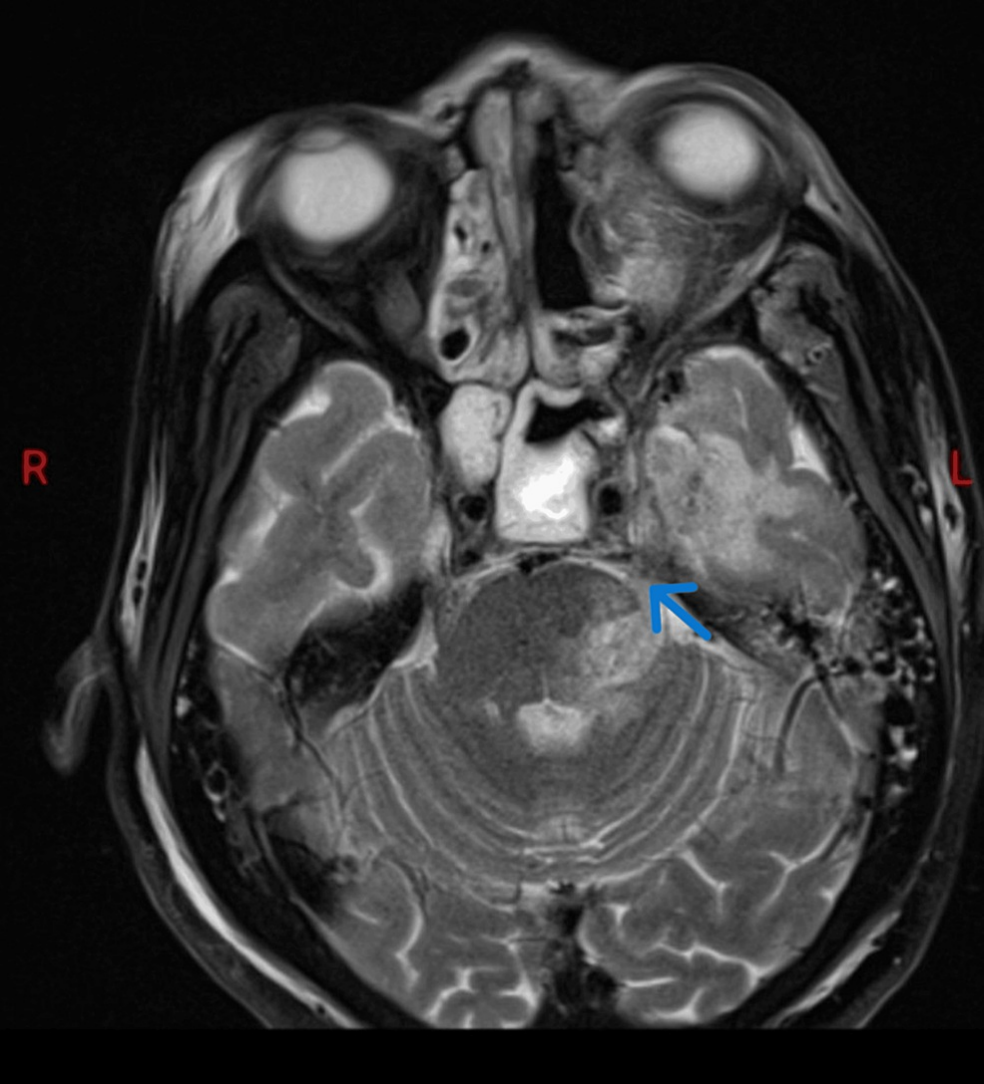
MRI brain T2 axial image shows thickening of the cisternal and cavernous portions of left trigeminal nerve (blue arrow) associated with T2 hyperintensity in the left hemipons and left middle cerebellar peduncle.
